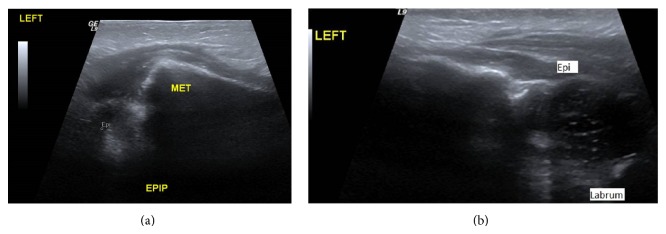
(a) Longitudinal sonogram of the left shoulder demonstrates separation of the left humeral epiphysis (epi) from the metaphysis (met). (b) Transverse sonogram of the left shoulder shows left humeral epiphysis within the confines of the labrum.
